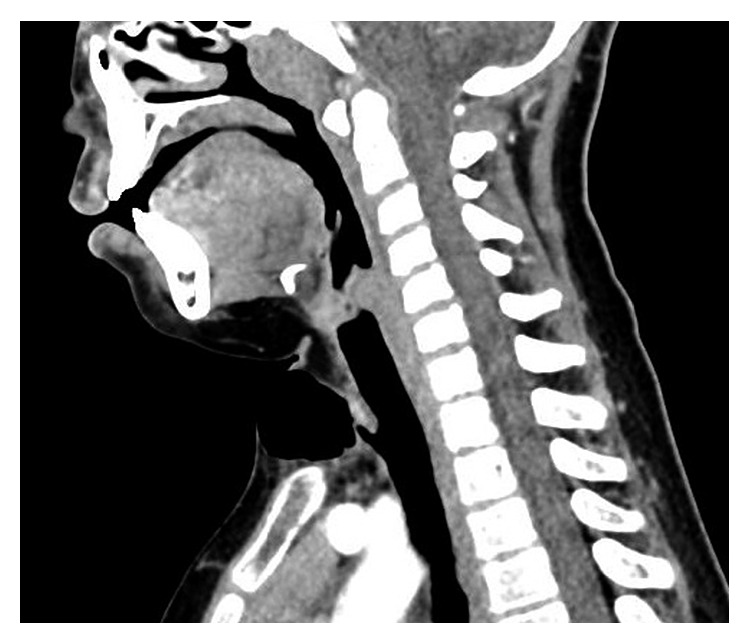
Sagittal plane CT neck demonstrating a small tracheal defect with subcutaneous air trapping to the level of the dermis.
